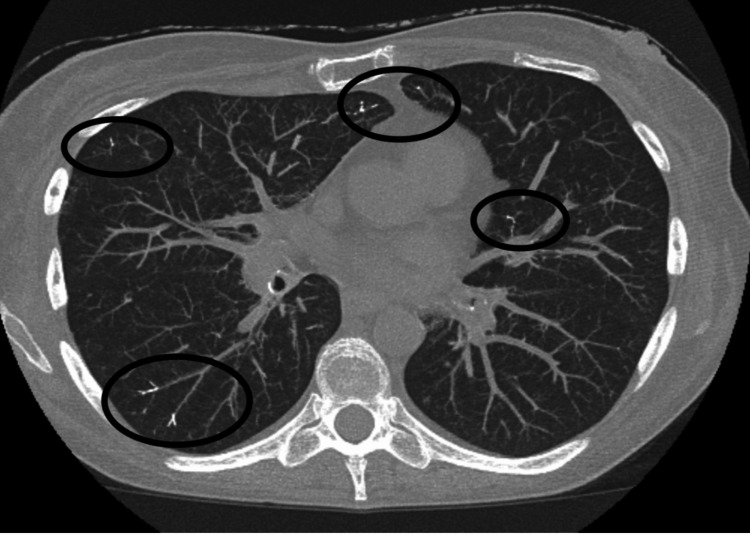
CT showing embolization of the lungs as indicated by the circles.
